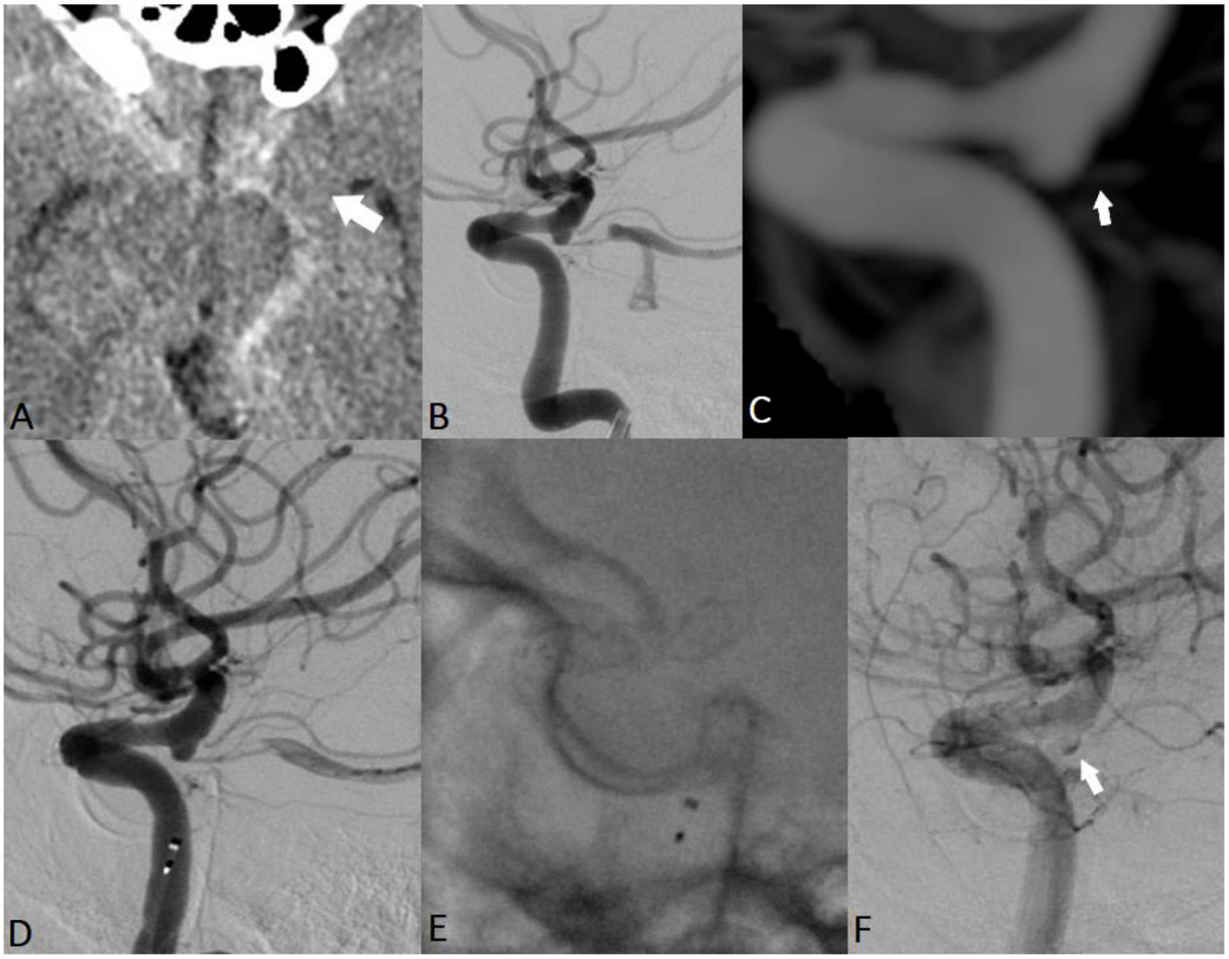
EVT of a ruptured PcomA ID in a 60-year-old man. Non-contrast cranial CT revealed a thin hemorrhage in the chiasmatic, interpeduncular, and left crural cisterns (A; white arrow). Subsequent repeated radiological examinations did not reveal any cerebrovascular pathology but confirmed the presence of an ID at the level of the left PcomA (B,C; white arrow). The assumed rupture site was the observed ID, and the patient received EVT (D,E). Noticeable contrast stagnation was observed in the PcomA ID after stent implantation (F).
